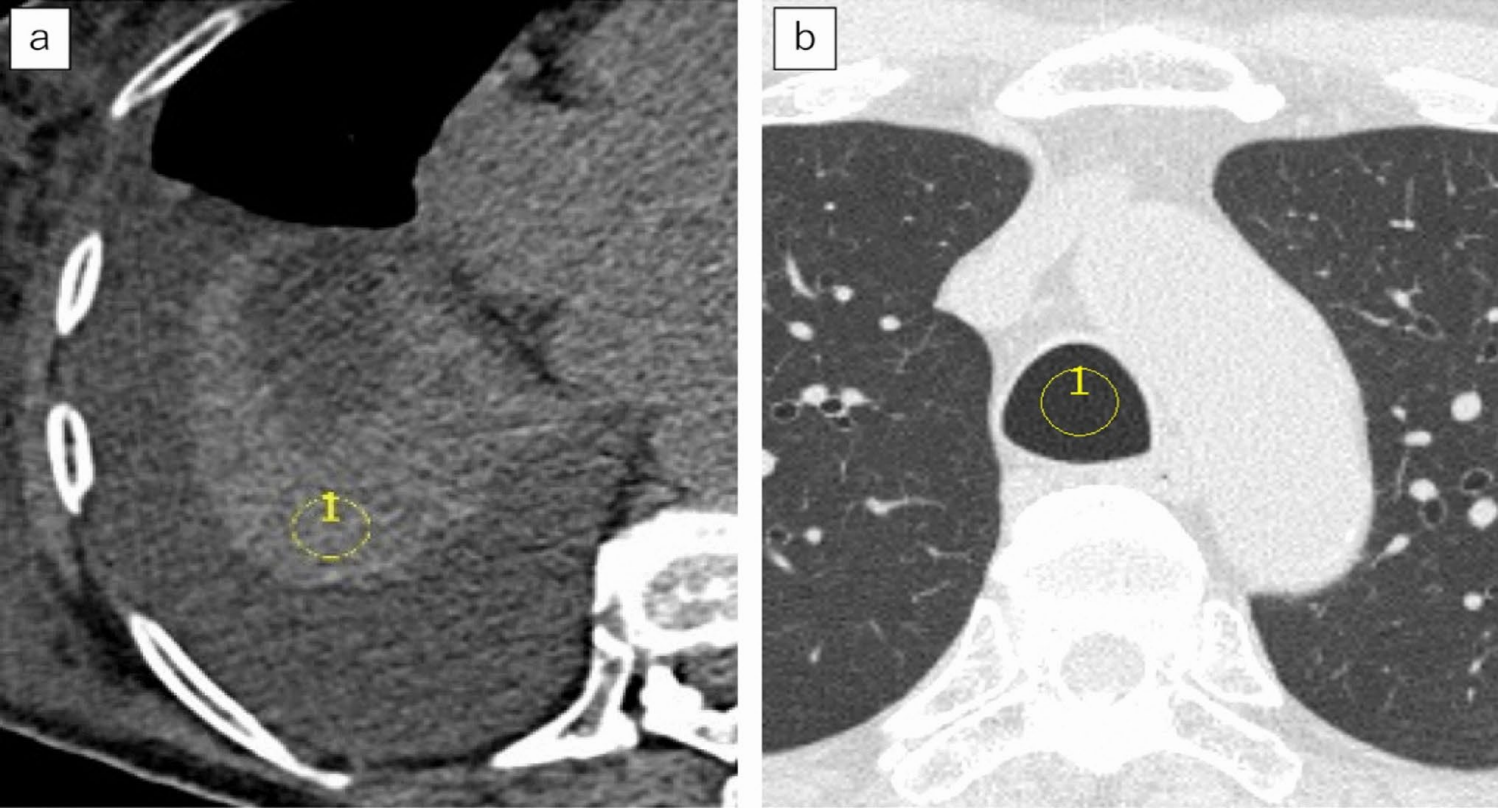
Image examples of CT number measurement in atelectasis and air in the trachea. a An example image of measuring CT numbers for atelectasis. A 91-year-old female. The yellow circle indicates the ROI. The central area of the atelectasis was measured, ensuring no pleural effusion or other structures were included. The left lung was similarly measured on another slice deemed optimal. b An example image of measuring CT numbers for air in the trachea. A 72-year-old male. The yellow circle indicates the ROI. Measurement was taken at a distance from the tracheal wall to avoid including it. *Note—*CT: computed tomography, ROI: region of interest
